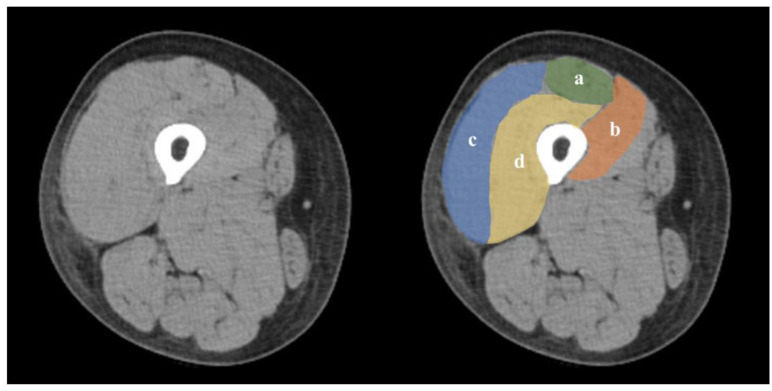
Measurement of quadriceps femoris using CT. Mid-thigh CT. a: Rectus femoris. b: Vastus medialis. c: Vastus lateralis. d: Vastus intermedius. CT, computed tomography.
